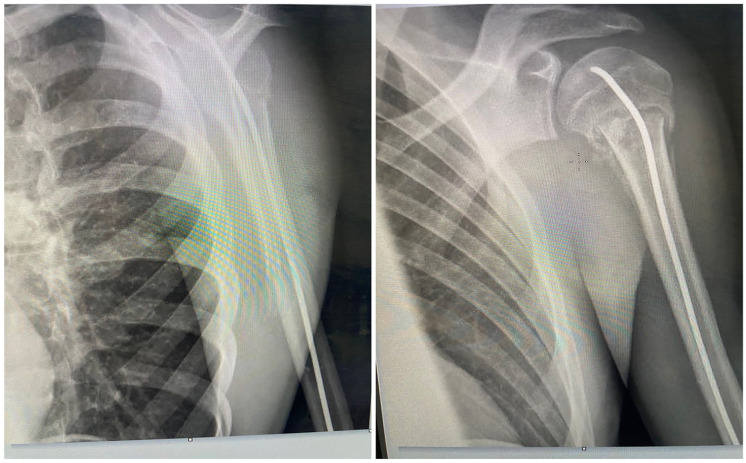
X-ray at 6-week follow-up with the SNT showing radiological union.
